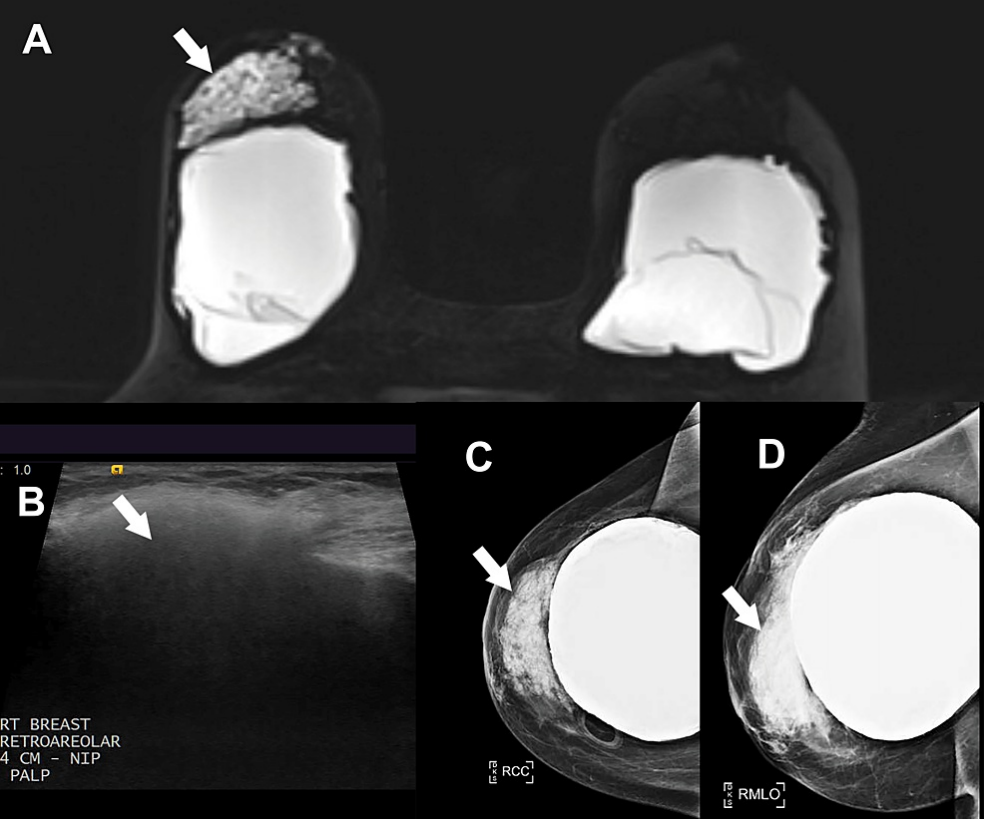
(A) Heterogeneous non-mass magnetic resonance imaging enhancement measuring 50 × 86 × 46 mm. (B) Ultrasound demonstrates heterogeneous echogenicity with dispersion of the ultrasound beam (“snowstorm appearance”). (C) Craniocaudal and (D) mediolateral oblique view mammogram images showing high density in the central right breast without any suspicious masses or calcifications.
